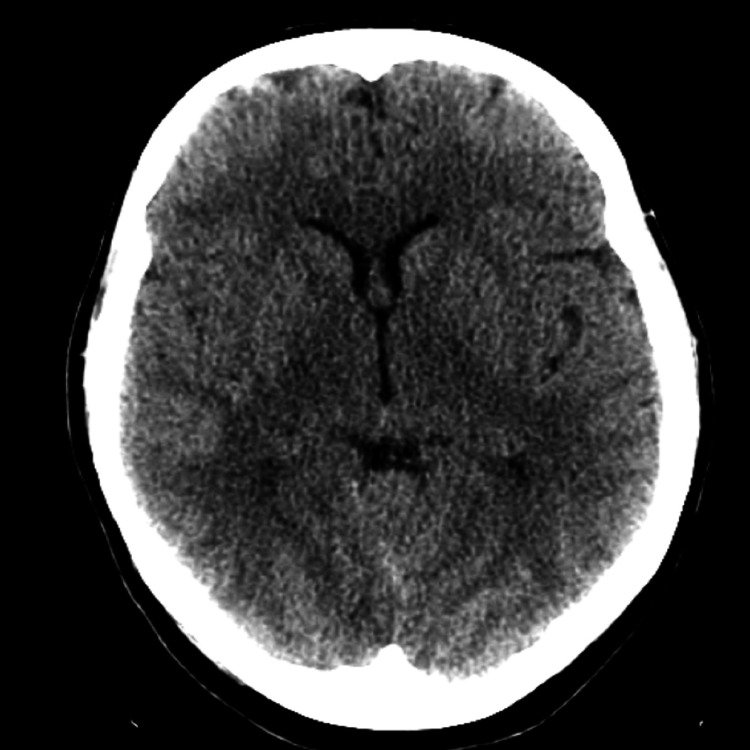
Repeat head CT scan showing resolved pneumocephalus.
